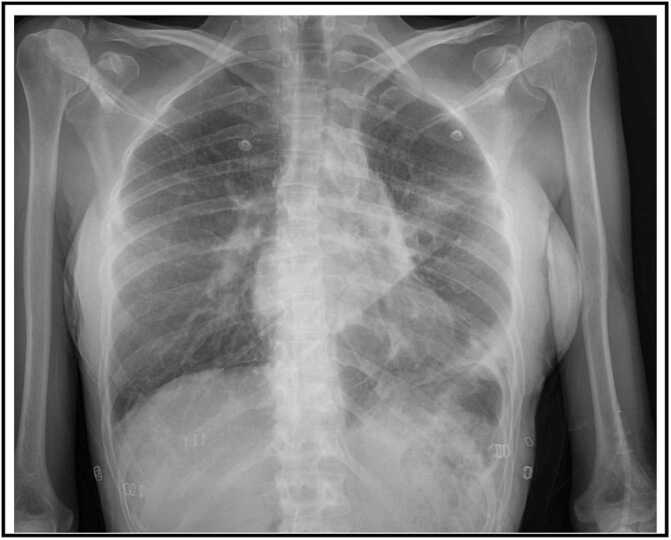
Chest X-ray 19 days post-transplant shows a vague shadowing in the left upper lobe.
